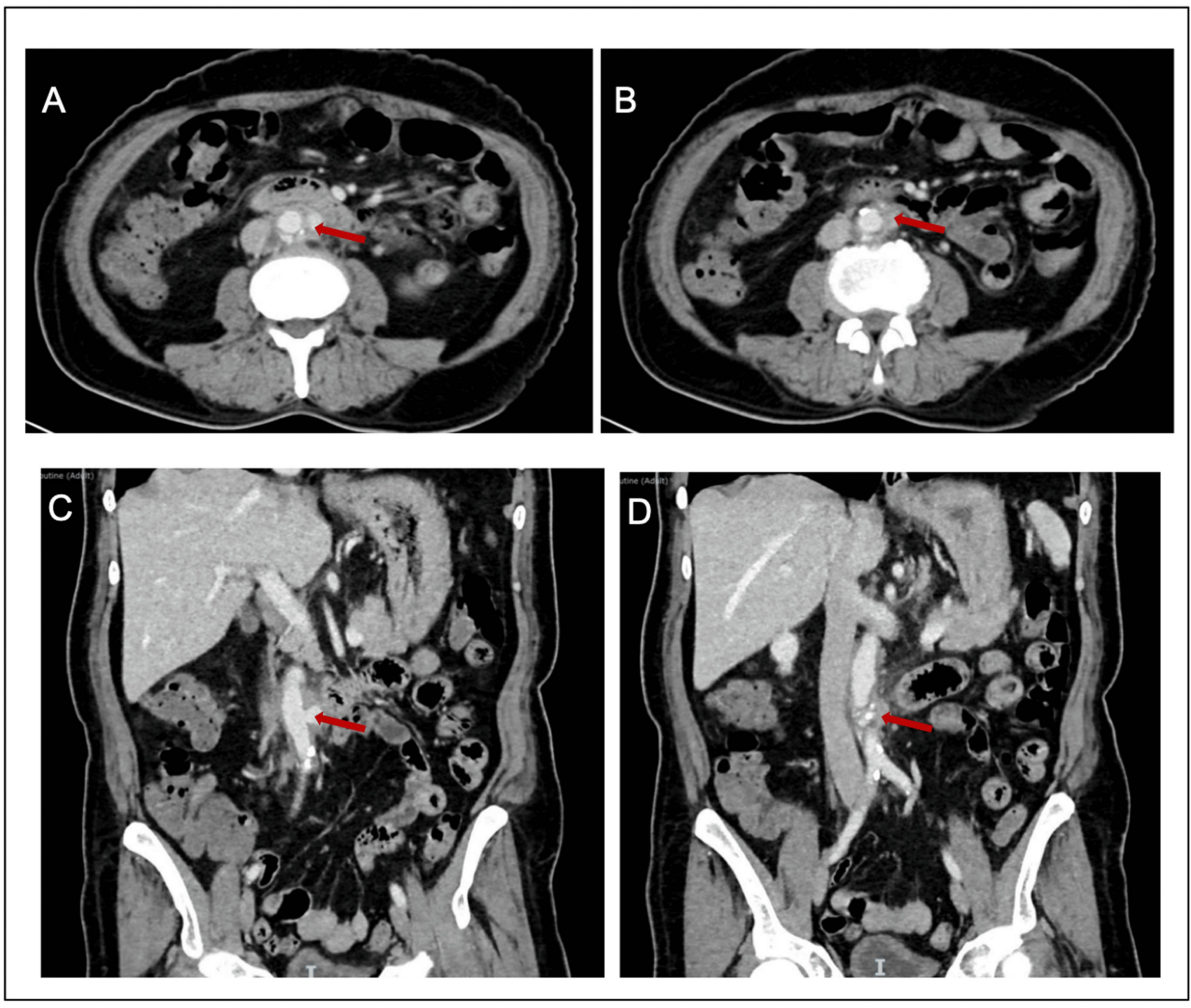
A and B: Contrast-enhanced computed tomography (CT) of the abdomen axial cuts showing infrarenal abdominal aorta pseudoaneurysm with an adjacent hematoma and fat stranding concerning for leakage. C and D: Contrast-enhanced CT of the abdomen coronal cuts showing infrarenal abdominal aorta pseudoaneurysm with an adjacent hematoma and fat stranding.
